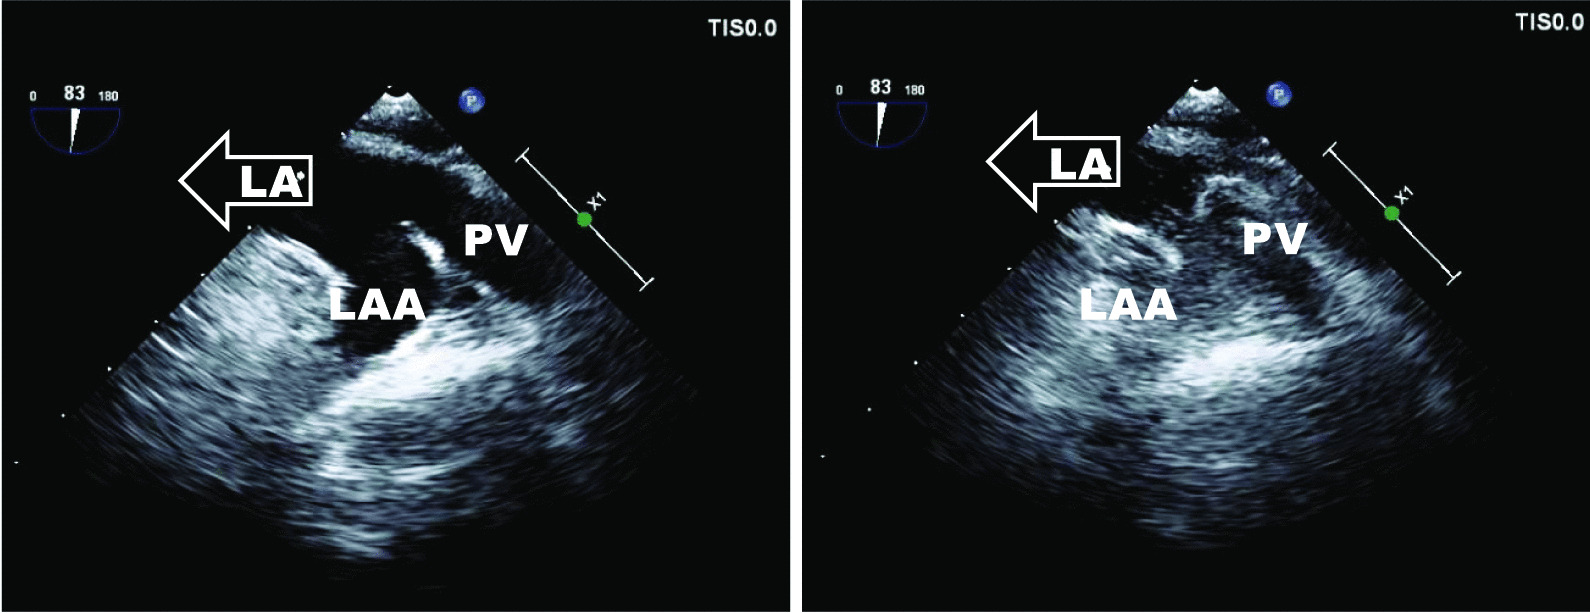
Transesophageal view at the junction of left atrial appendage (LAA) and left pulmonary veins (PV) before inflow into the left atrium (*LA*; left image). The contrast media inflow origins in the left atrial appendage where the opacification commences (right image)
